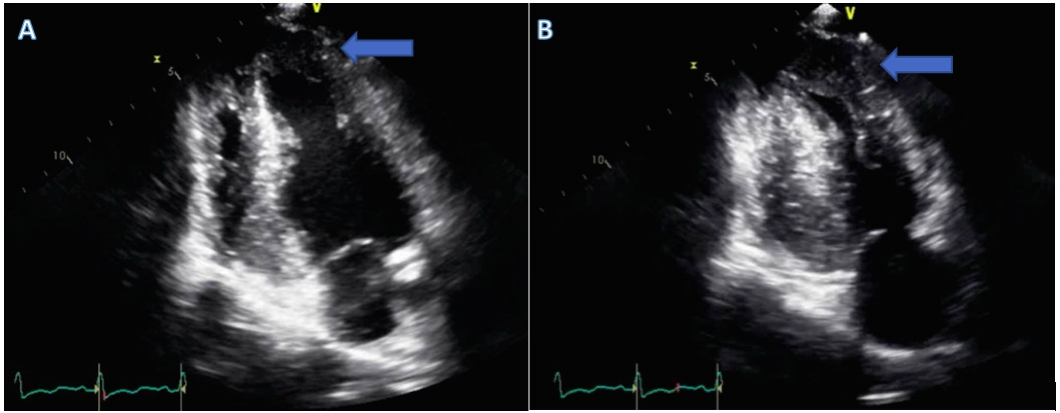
Echocardiogram: apical HCM is seen in diastole (A) and in systole (B) HCM - hypertrophic cardiomyopathy.
